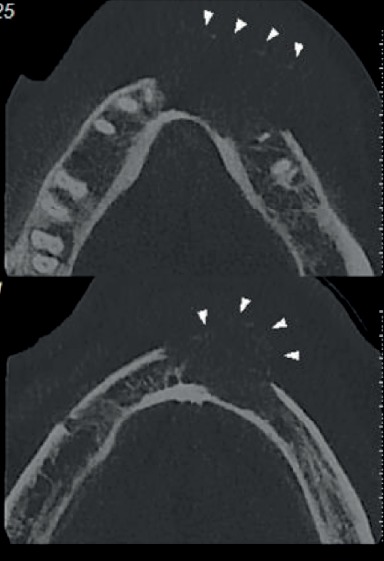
CBCT revealed a large destructive lesion with irregular borders associated with a soft tissue mass causing extensive perforation of the buccal cortical plate.
